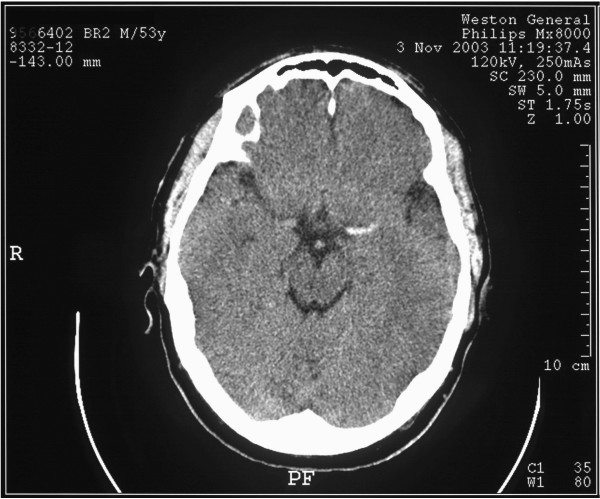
Computed tomography scan of the brain at admission.
